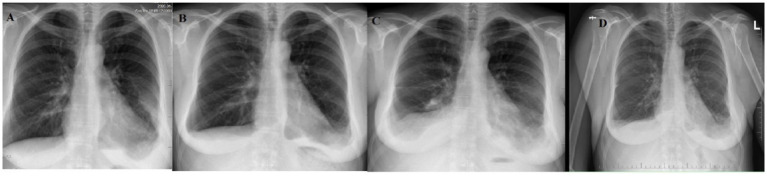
Patient No. 3’s chest X-ray examinations. (A) PA chest X-ray image at the time of treatment initiation shows single opacities in the lower field of the right lung with a small amount of fluid in the right pleural cavity and fluid in the left pleural cavity. (B) PA chest X-ray image after 1 year of sirolimus treatment shows partial regression of fluid in the left pleural cavity. (C) PA chest X-ray image after 2 years of sirolimus treatment shows an increased amount of fluid in the left pleural cavity, reappearance of fluid in the right pleural cavity, and increased consolidation in the lower field of the right lung. (D) PA chest X-ray image after 5 years of sirolimus treatment shows partial regression of fluid in the right pleural cavity and stabilization of fluid in the left pleural cavity.
